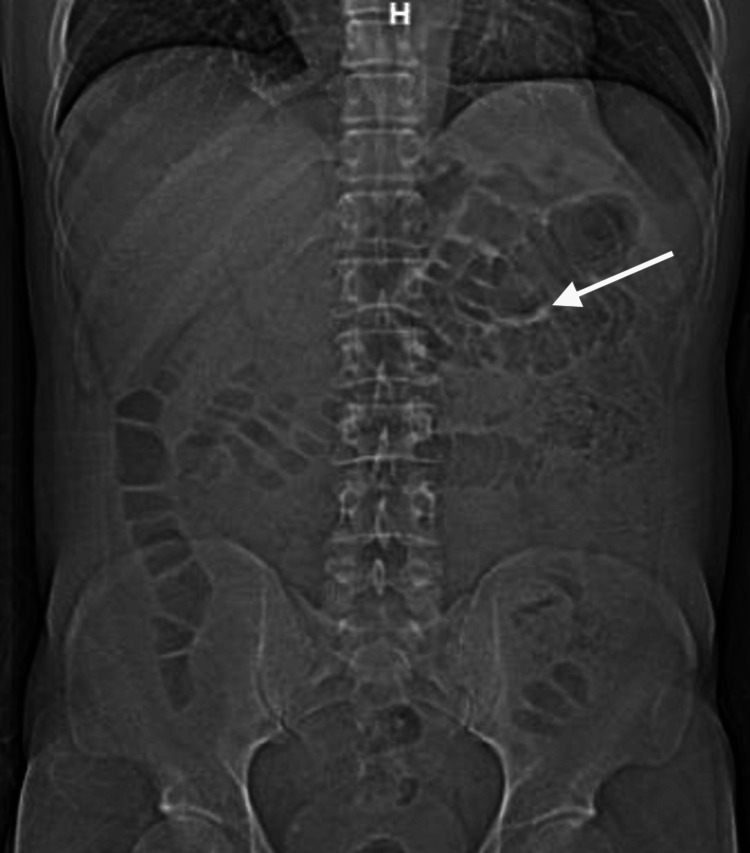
A CT scan of the abdomen and pelvis (anteroposterior (AP)) view showing bowel wall thickening suggestive of ileocolitis
